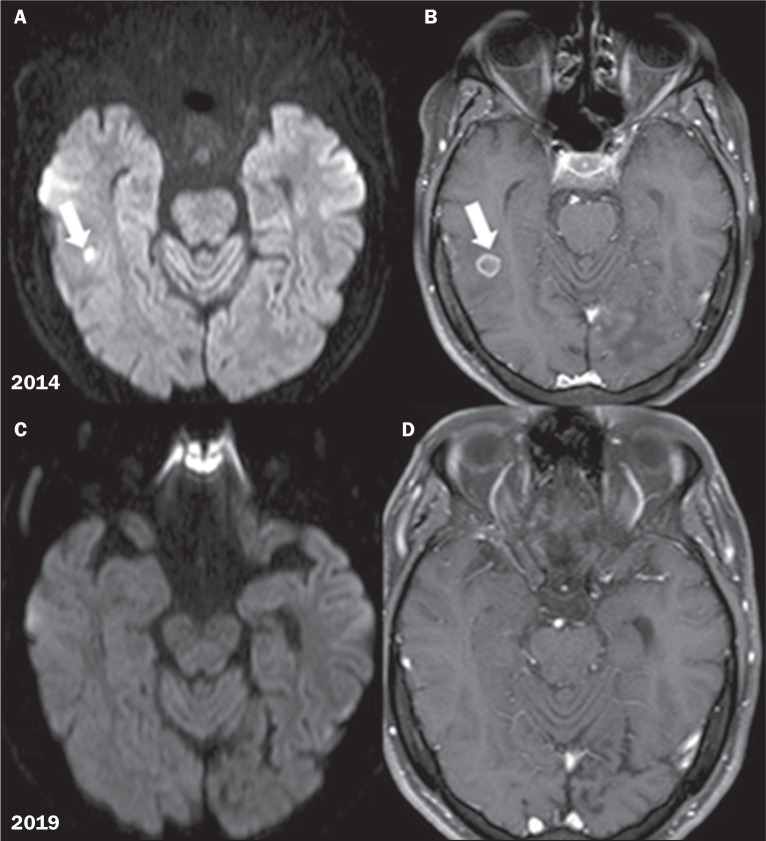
Diffusion-weighted imaging (DWI) analysis. A: Axial DWI showing central
